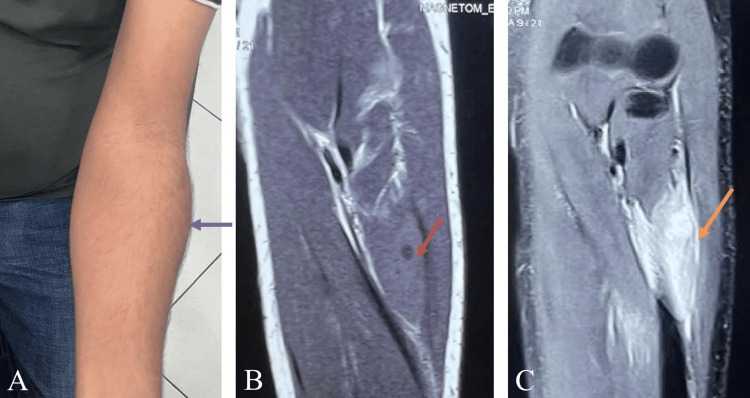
Left forearm A) Left forearm marked swelling near elbow (purple arrow). B) MRI T1-weighted image of left forearm shows cystic lesion with hypointense center with surrounding hyperintense area suggestive of scolex (red arrow). C) Intense contrast enhancement in left forearm muscles (orange arrow).
